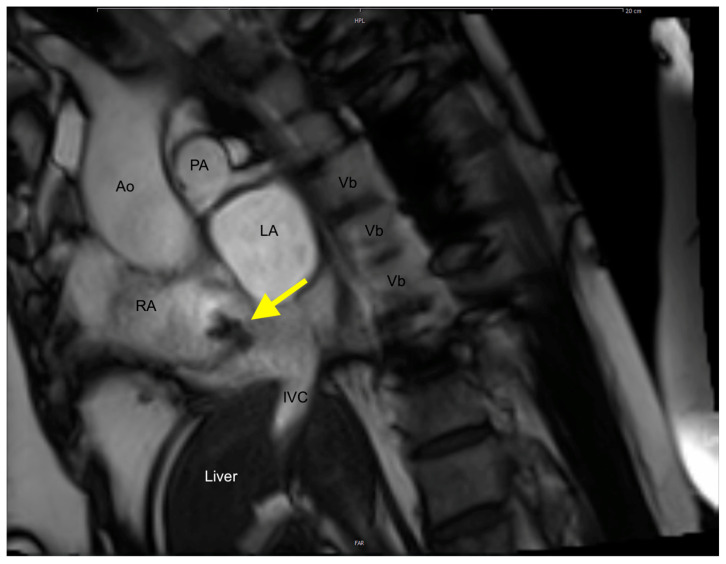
Cardiac MRI demonstrating the CAT in the right atrium (yellow arrow). (Ao, Aorta; IVC, Inferior Vena Cava; LA, Left atrium; PA, Pulmonary artery; RA, Right Atrium; Vb, Vertebra).
